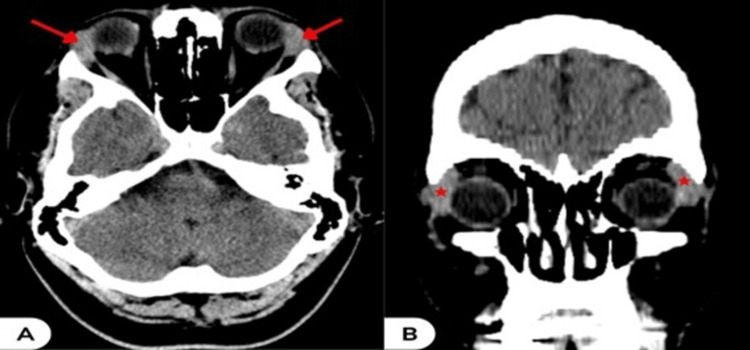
(A) Axial view head CT showing bilateral lacrimal gland enlargement (red arrows); (B) Coronal view (red stars)
